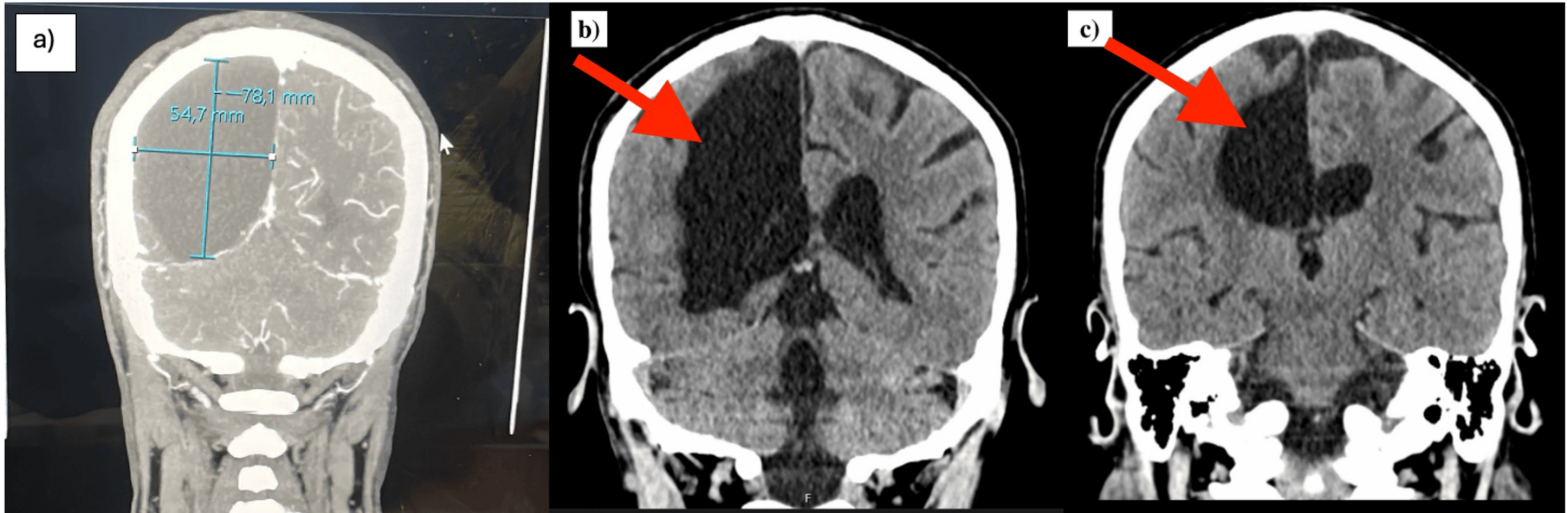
Coronal brain computed tomography images demonstrating the size and location of the right parieto-occipital arachnoid cyst. a: coronal brain CT image showing measurement of the right parieto-occipital arachnoid cyst in maximal dimensions (54.7 x 78.1 mm); b,c: coronal views showing the right parieto-occipital cyst (arrows) with preservation of midline structures and absence of surrounding edema.
